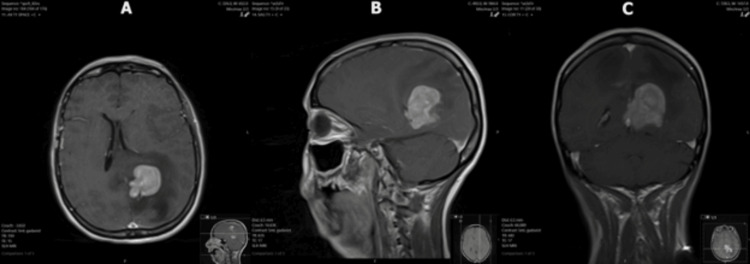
T1 post-contrast MRI images demonstrating significant interval enlargement of the left cingulate gyrus/frontoparietal lesion with surrounding vasogenic edema and new left-to-right midline shift (A) Axial, (B) sagittal, and (C) coronal views of the left cingulate gyrus/frontoparietal lesion, which is enlarged from prior imaging, with new vasogenic edema, mass effect, and mild left transtentorial herniation. MRI: magnetic resonance imaging
